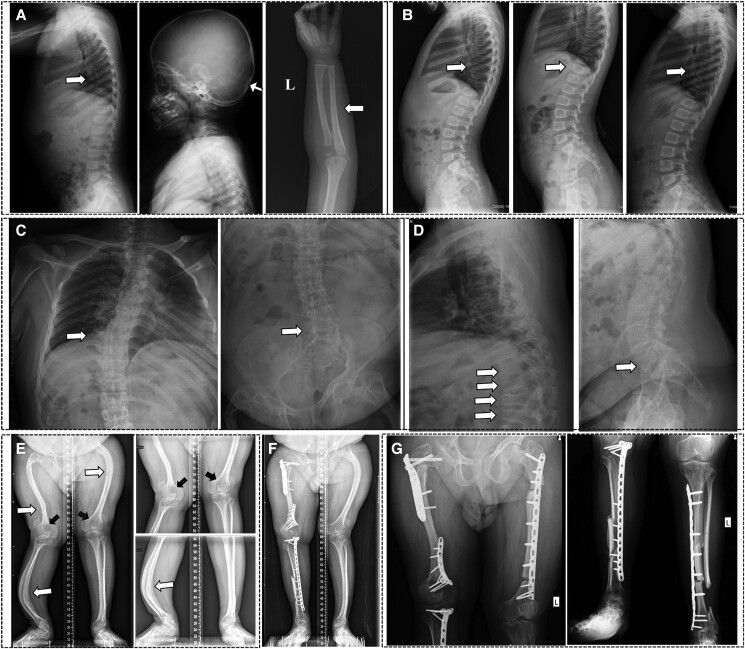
Radiological findings of the patients with *CRTAP* mutations. (A) Radiographs of thoracolumbar and skull and left ulna and radius bone of patient 1 at baseline. (B) Radiographs of thoracolumbar of patient 1 after 1 year, 3 years of zoledronic acid treatment and after discontinuation of zoledronic acid for 1 year. (C) Anteroposterior view of thoracic and lumbar vertebra of patient 2 at baseline. (D) Lateral film of thoracic and lumbar vertebra of patient 2 at baseline. (E) Radiographs of lower extremities of patient 2 at baseline. (F) Radiographs of lower extremities of patient 2 at 5 months after the first surgery. (G) Radiographs of bilateral femurs, tibia, and fibula of patient 2 at 5 months after the second surgery.
